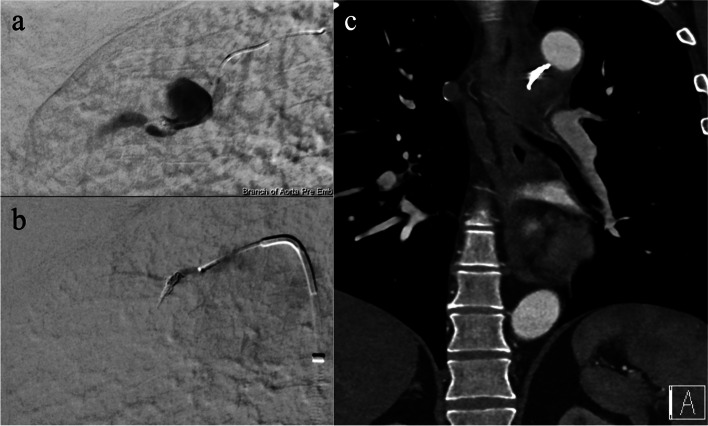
Bronchial artery pseudoaneurysm pre and post embolization. a Selective angiogram via a microcatheter in the right bronchial artery showing the pseudoaneurysm. b Selective angiogram post coil embolization demonstrating no residual filling in the pseudoaneurysm. c Follow-up CT angiogram one day post procedure demonstrating successful exclusion of pseudoaneurysm
